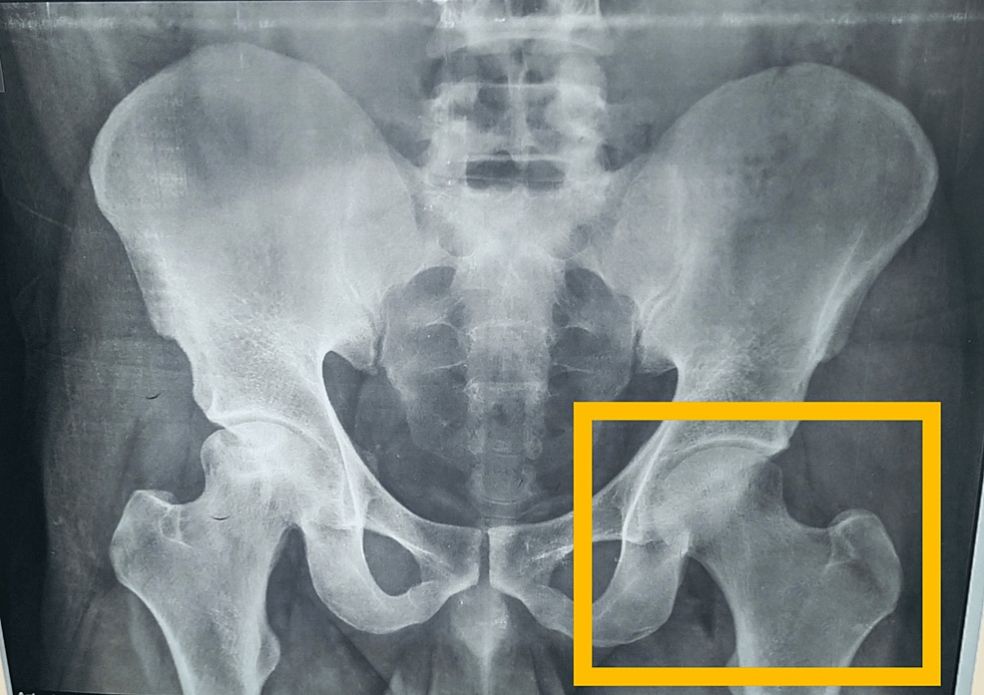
X-ray showing PA view of the hip joint with sclerotic and erosive changes in the left head of the femur
